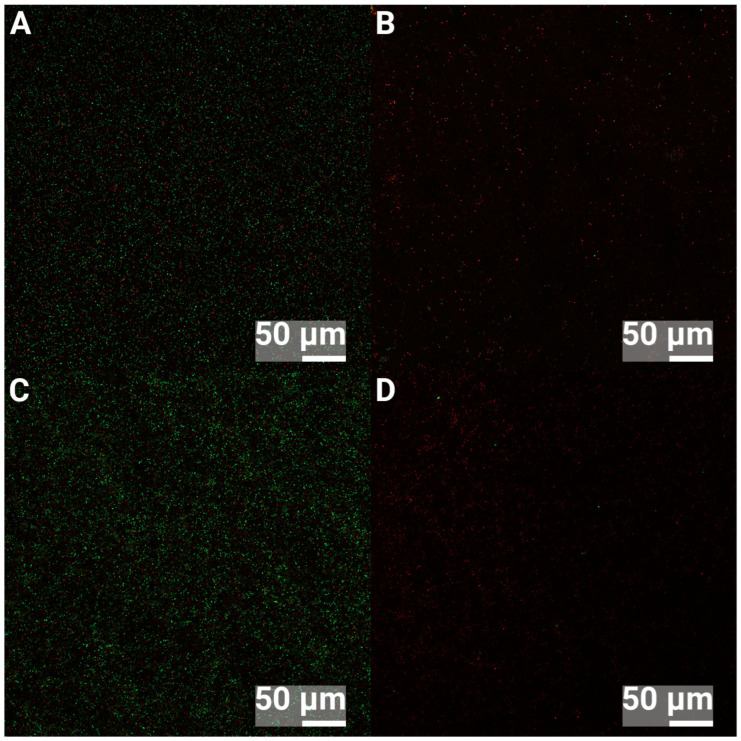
CLSM images of 24 h biofilms of *S. epidermidis*. (A) shows Ti and no ultrasound treatment, (B) shows Ti and 5 min ultrasound treatment, (C) shows nHA and no ultrasound treatment and (D) shows nHA with 5 min ultrasound treatment. Green bacteria are alive, and red bacteria are dead.
